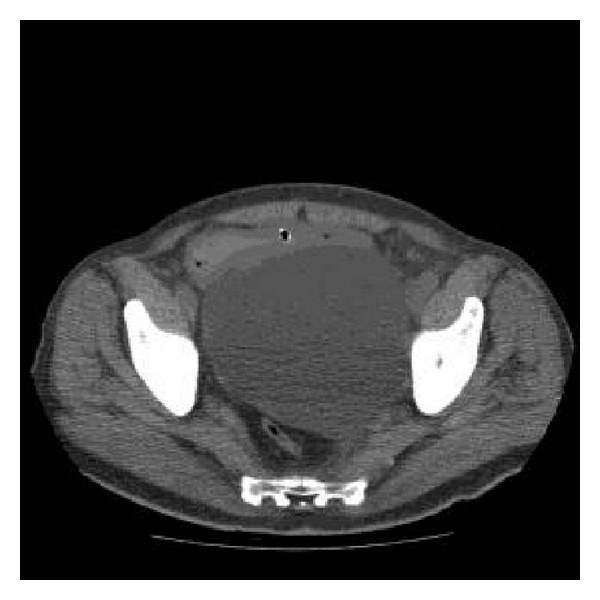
Abdominal CT scan: retrovesical 14 × 14 × 12 cm cystic mass and bilateral obstructive uropathy.
